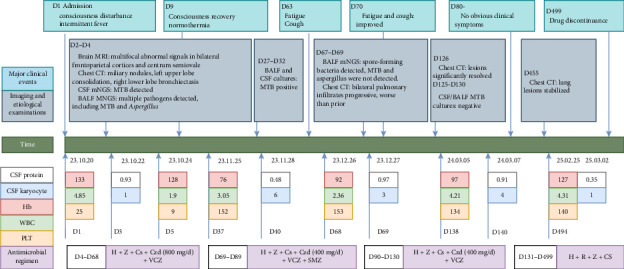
Overview of key clinical events, examination results, and treatment regimens. Abbreviations: BALF: bronchoalveolar lavage fluid; Cs: cycloserine; CSF: cerebrospinal fluid; Czd: contezolid; D: patient day(s); H: isoniazid; Hb: hemoglobin; mNGS: metagenomic next-generation sequencing; MRI: magnetic resonance imaging; MTB: *Mycobacterium tuberculosis*; PLT: platelets; R: rifampicin; RBC: red blood cells; SMZ: sulfamethoxazole-trimethoprim; WBC: white blood cells; VCZ: voriconazole; Z: pyrazinamide.
